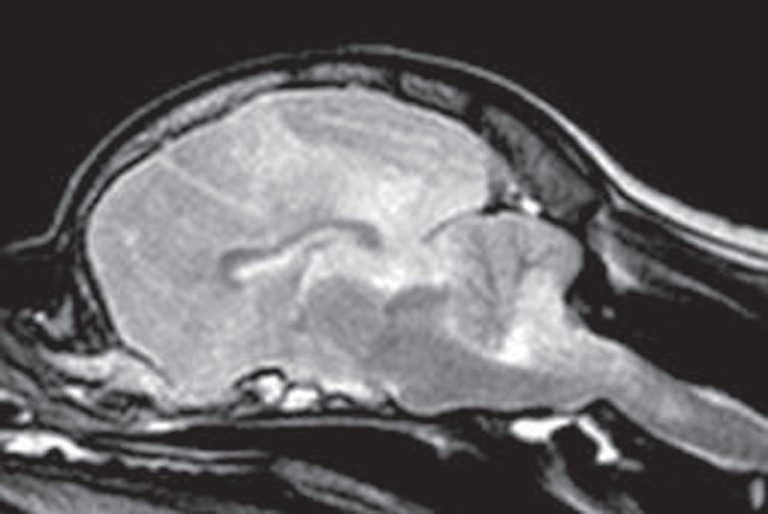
Sagittal T2-weighted magnetic resonance image (0.5 T) of a Pug (9 months, female) with necrotizing meningoencephalitis (NME). Caudal transtentorial herniation of the occipital lobes and foramen magnum herniation of the cerebellum caused by significant swelling of the prosencephalon. This may explain the sudden death of some Pugs with NME.
